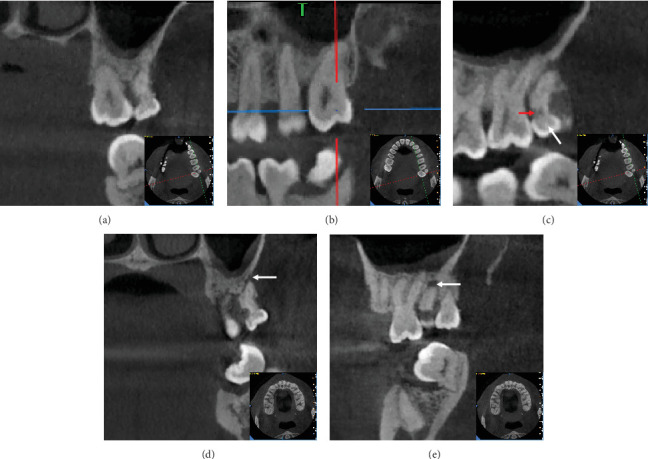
(a) Coronal view of the patient's CBCT scan showing the maxillary third and fourth molars in the left maxillary quadrant. (b, c) Sagittal views of the maxillary (b) third molar and (c) fourth molar in the left maxillary quadrant. (c) The red arrow indicates an area of decreased density on the mesial aspect of the fourth molar root, while the white arrow points to the left maxillary fourth molar. (d, e) White arrows highlight areas of decreased density in the periapical region of the fourth molar root.
